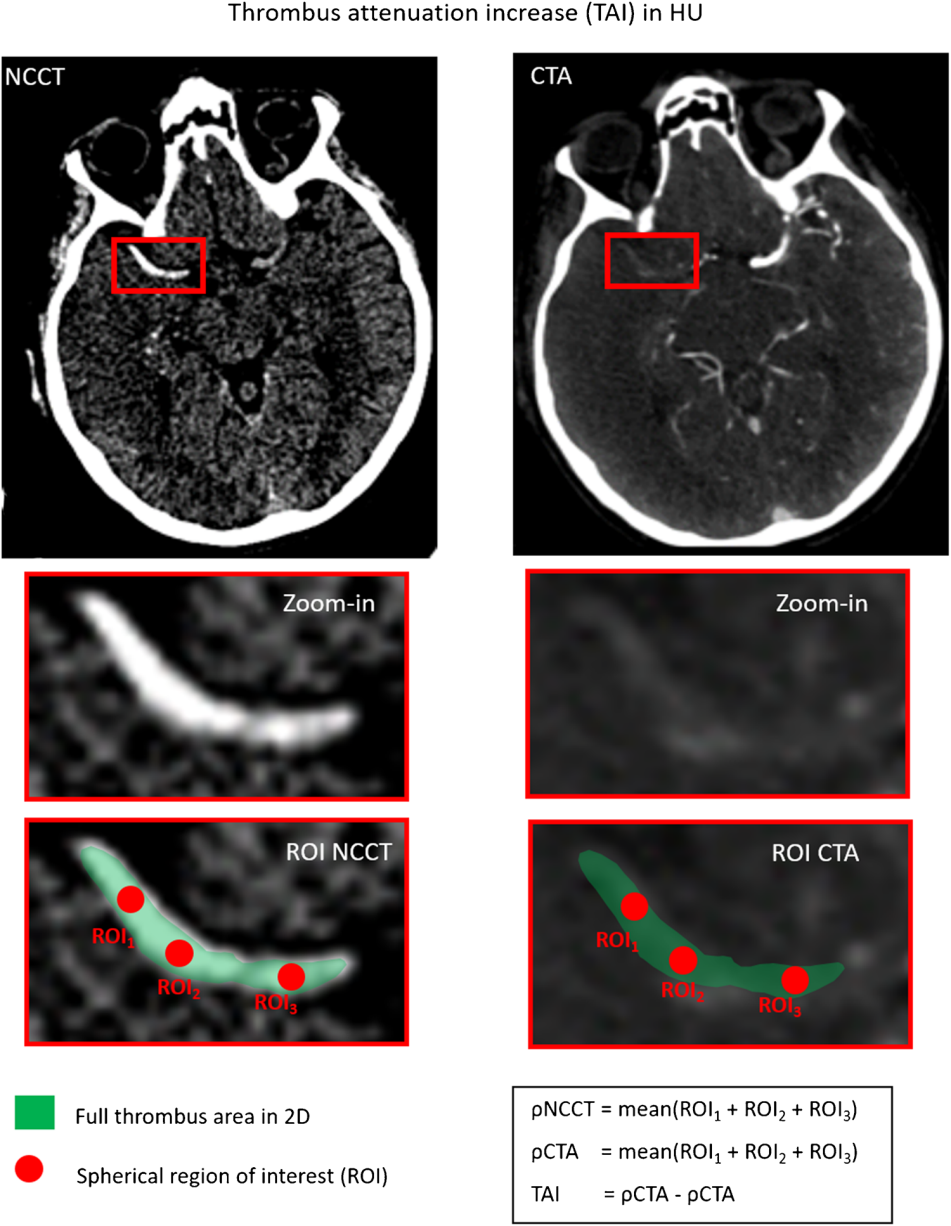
Calculation of thrombus perviousness as thrombus attenuation increase (TAI) between non-contrast CT (NCCT) and CT angiography (CTA). As this method is the most widely used in the literature, it is referred to as the standard perviousness (TAI_standard_) in this review
